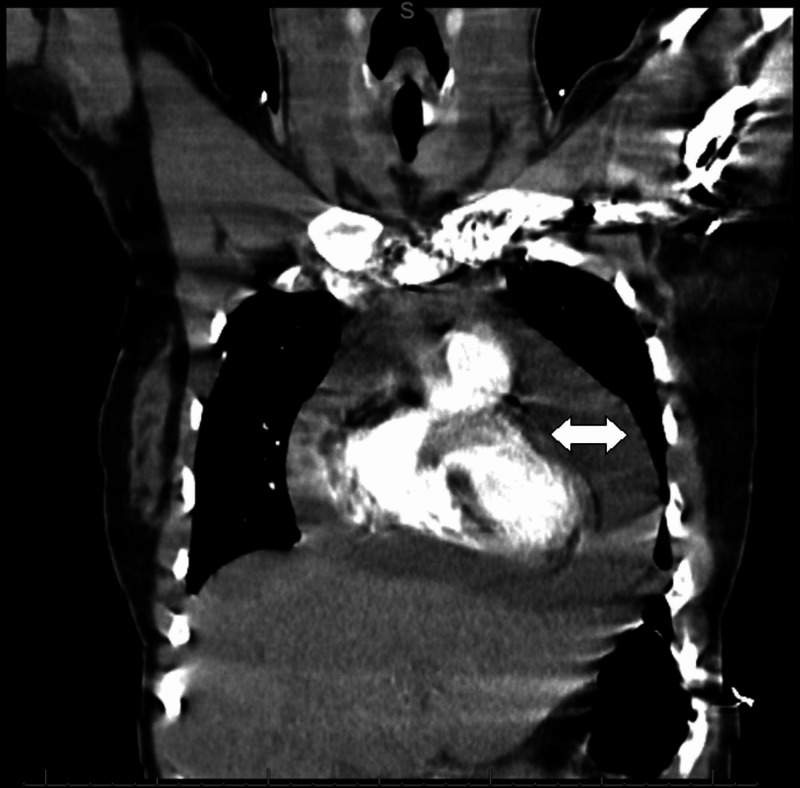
Coronal view of the computed tomography angiogram of the chest revealing a large circumferential pericardial effusion
